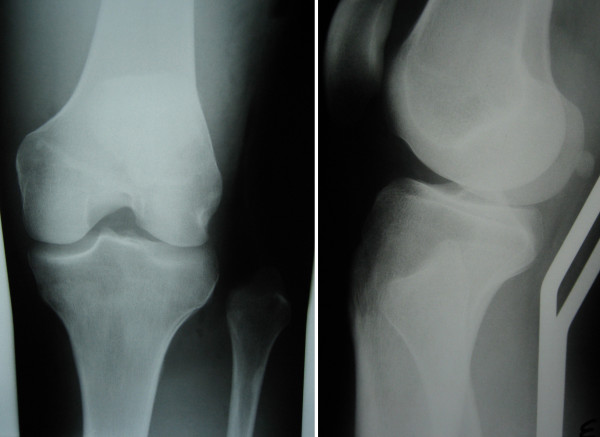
Anteroposterior and Lateral Knee X-ray. The proximal tibiofibular dislocation.
